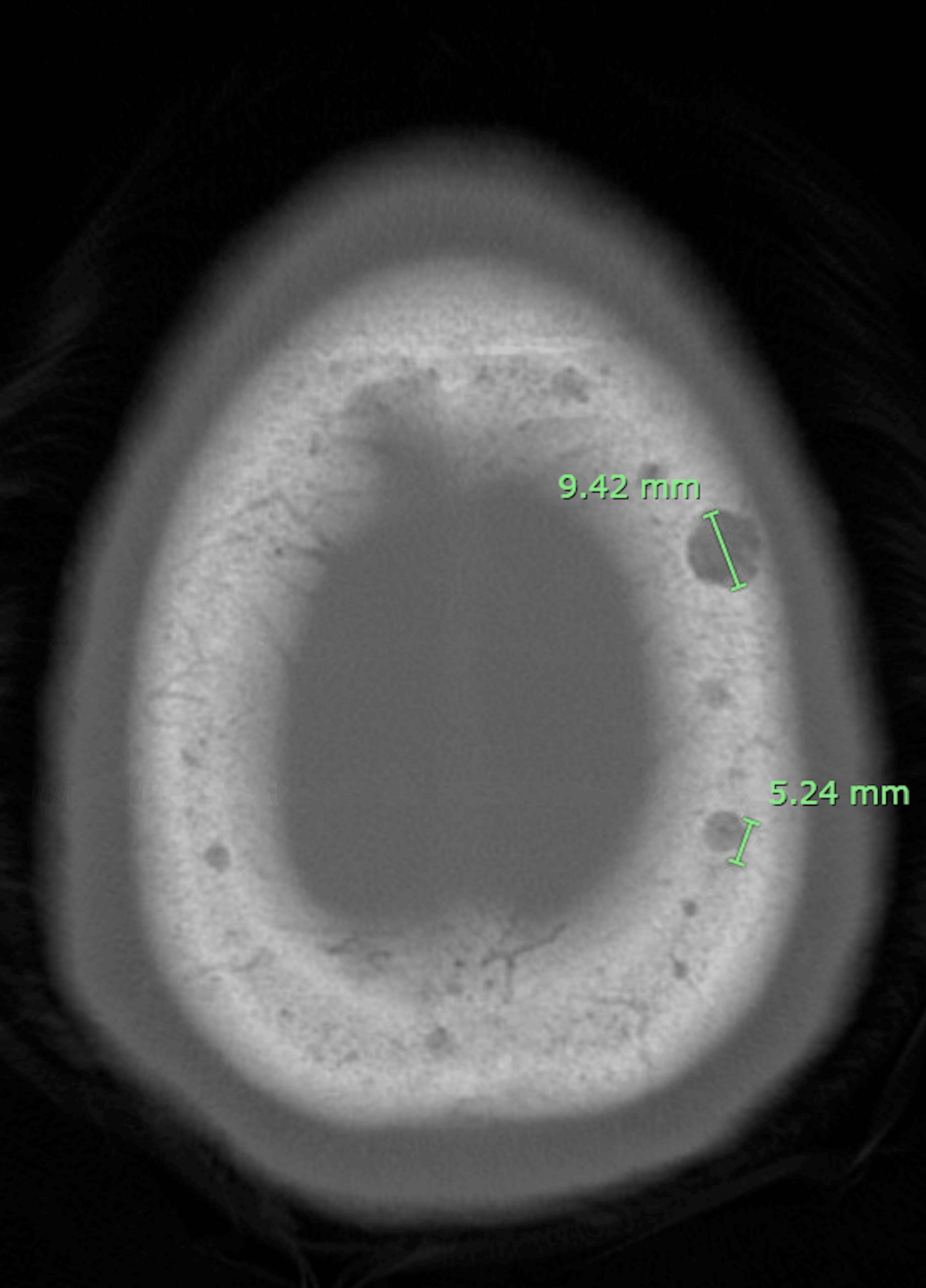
CT shows lytic lesions present bilaterally in the calvarium near the calvarial apex. The largest is on the right side, measuring 9.42 mm in diameter. CT: computed tomography
